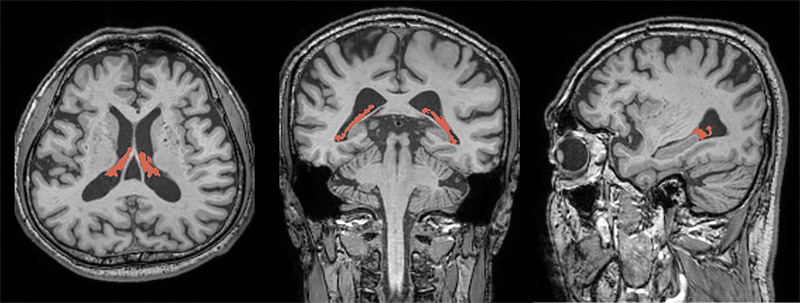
Choroid plexus segmentation. Representative images showing choroid plexus segmentation (red) overlaid on three-dimensional T1-weighted magnetic resonance images in the axial (left), coronal (center), and sagittal (right) planes.
